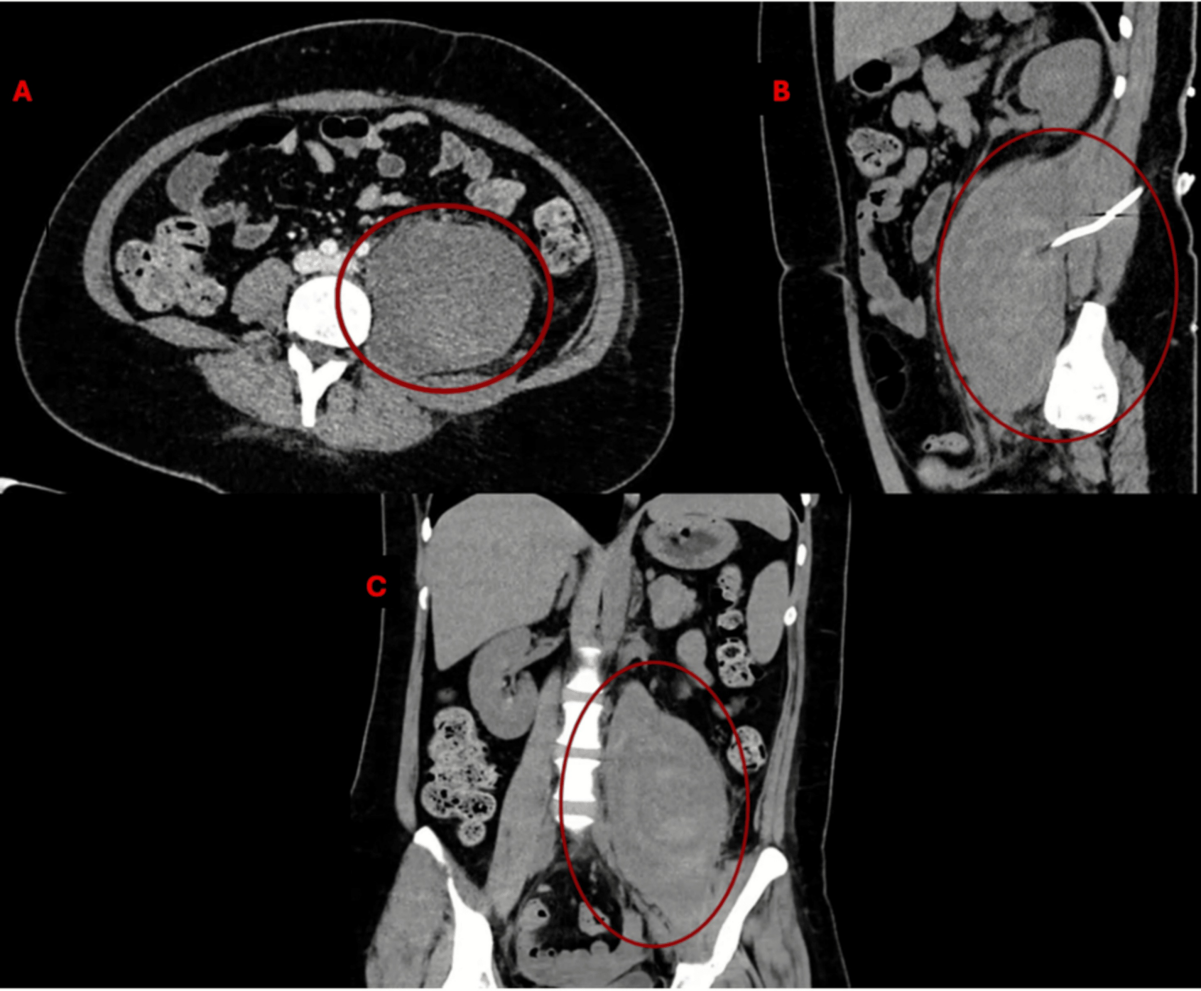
Hematoma in the iliopsoas noted via initial contrast-enhanced computed tomography (CT) scan Cross-section (A) and sagittal section (B) demonstrating correct placement of drainage via radiointervention. Pane C showcases the coronal section.
